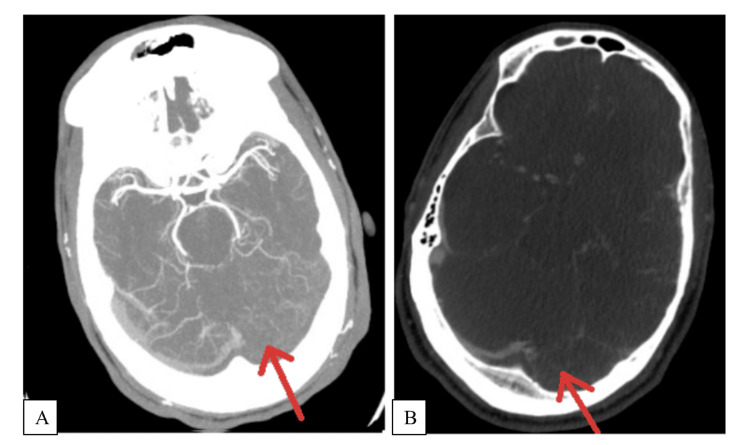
CTA and CTV showing CVST. (A) CTA head and neck showing absent contrast opacification of the left transverse sinus (arrow). (B) CTV showing dural venous sinus thrombosis involving the left transverse sinus (arrow). CTA: CT angiography; CTV: CT venogram; CVST: cerebral venous sinus thrombosis.
